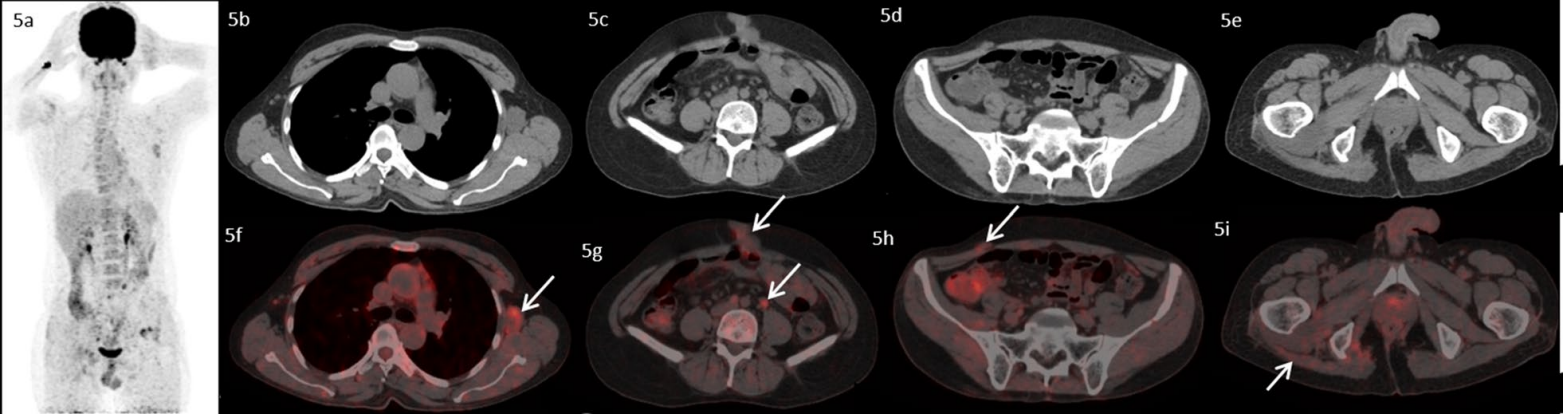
18F-FDG PET/CT images of the patient ten months after initiation of therapy with erlotinib. MIP image (5a) transaxial CT images (5b–5e) and fused PET/C images (4f–4i) show no significant interval change in size of the left axillary lymph node, anterior abdominal wall lesion and omental/mesenteric deposits and lesion near left ischial tuberosity (arrows) with mild reduction in metabolic activity, overall findings suggestive of stable disease
